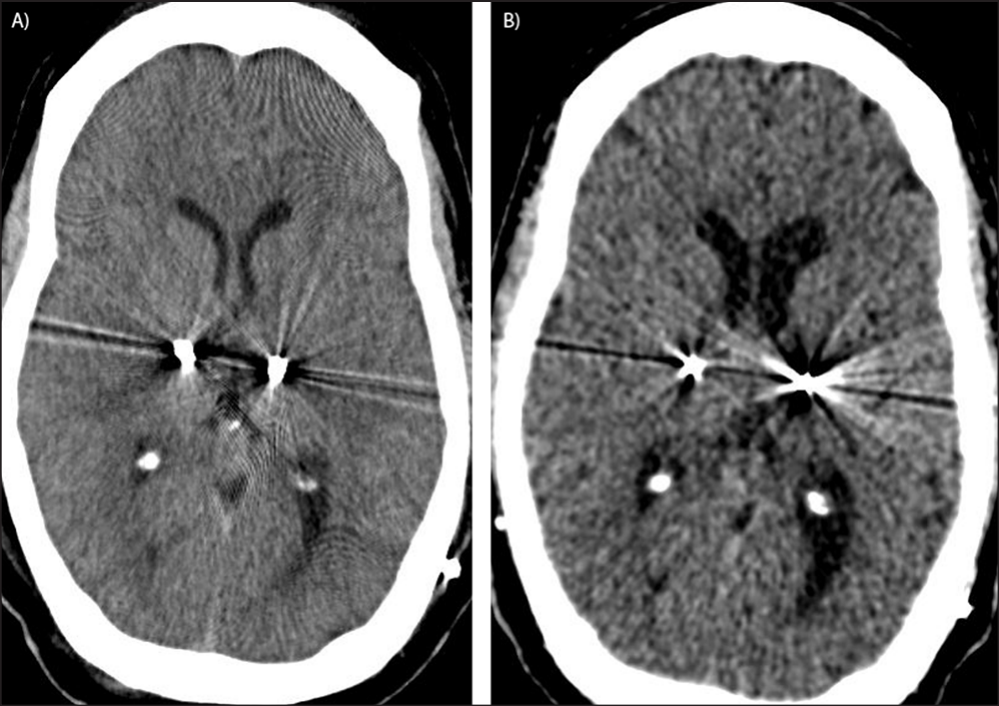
Changes in noncontrast head CT over time. Initial postoperative CT in 2014 (A) was normal, with bilateral VIM-DBS leads in place. Recent CT in 2019 (B) shows increased ventricular size and subtle left > right hemispheric atrophy.
